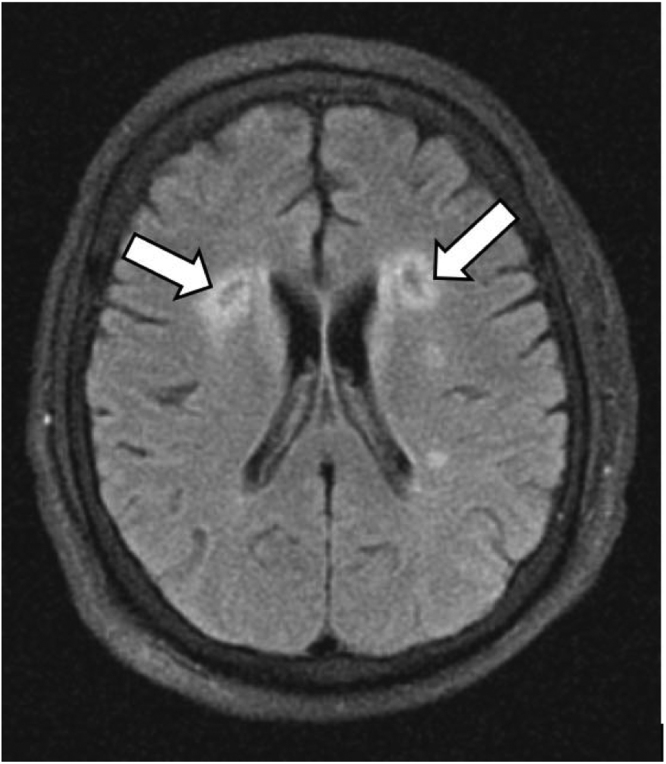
Follow-up non-enhanced MRI of the brain; Axial FLAIR sequence demonstrates central encephalomalacia in the lesions within the frontal lobe (White arrows).
